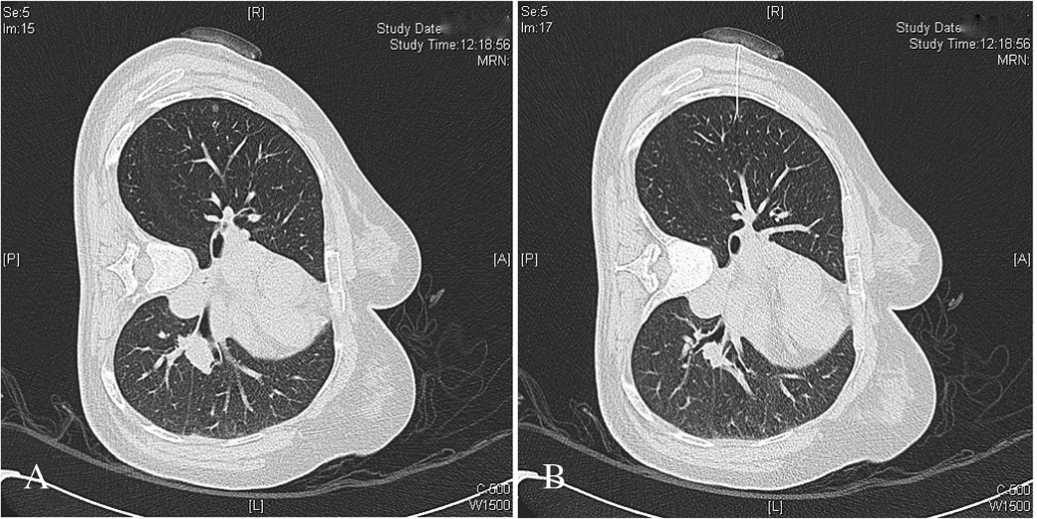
CT images during localization. (A) CT image showing the subpleura pulmonary lesion in the S2b of the right upper lobe; (B) CT image showing mild pneumothorax and the tip of wire 4 mm below the lesion.
